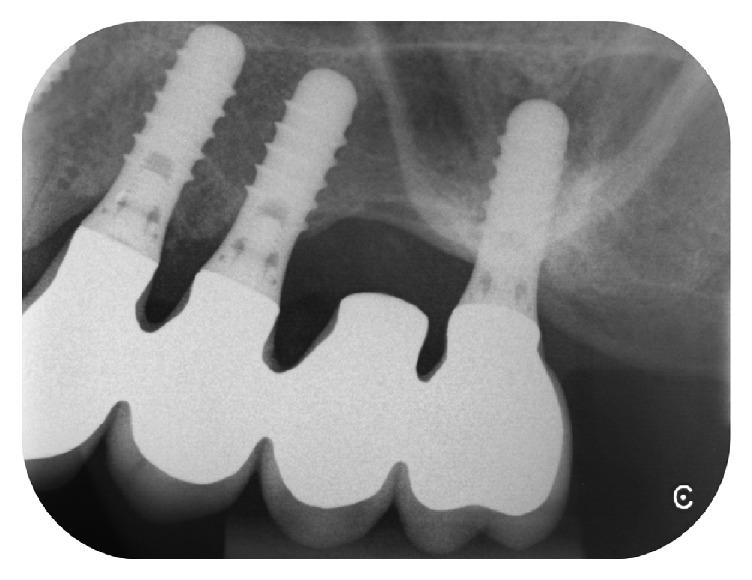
Two years after the closure of OAF; the radiographic control showed a stable crestal bone level.
